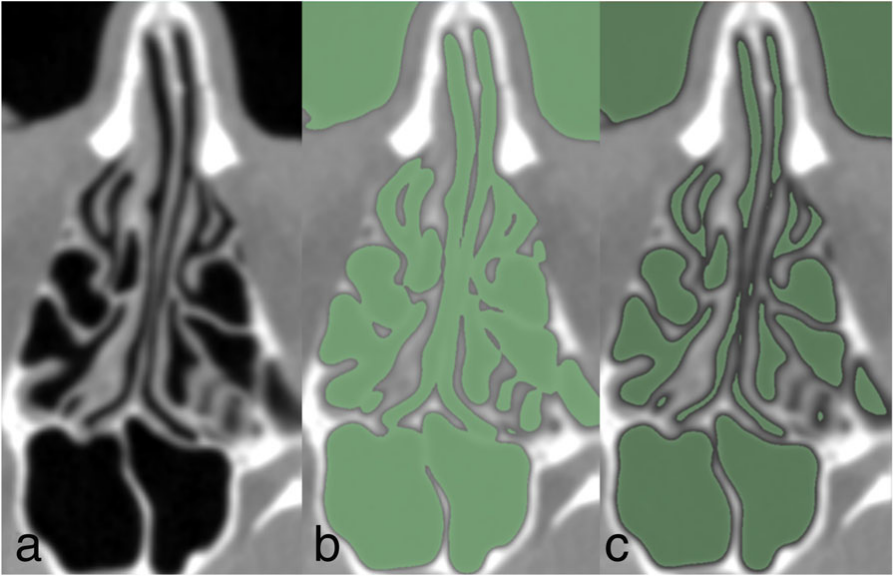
Nasal cavity segmentation. a Original, b over- and c under-thresholded segment of an axial CT image of a nasal cavity and adjacent sinuses. The green colour highlights the segmented area. The reference scale bar length in the lower right corner is 1 cm
